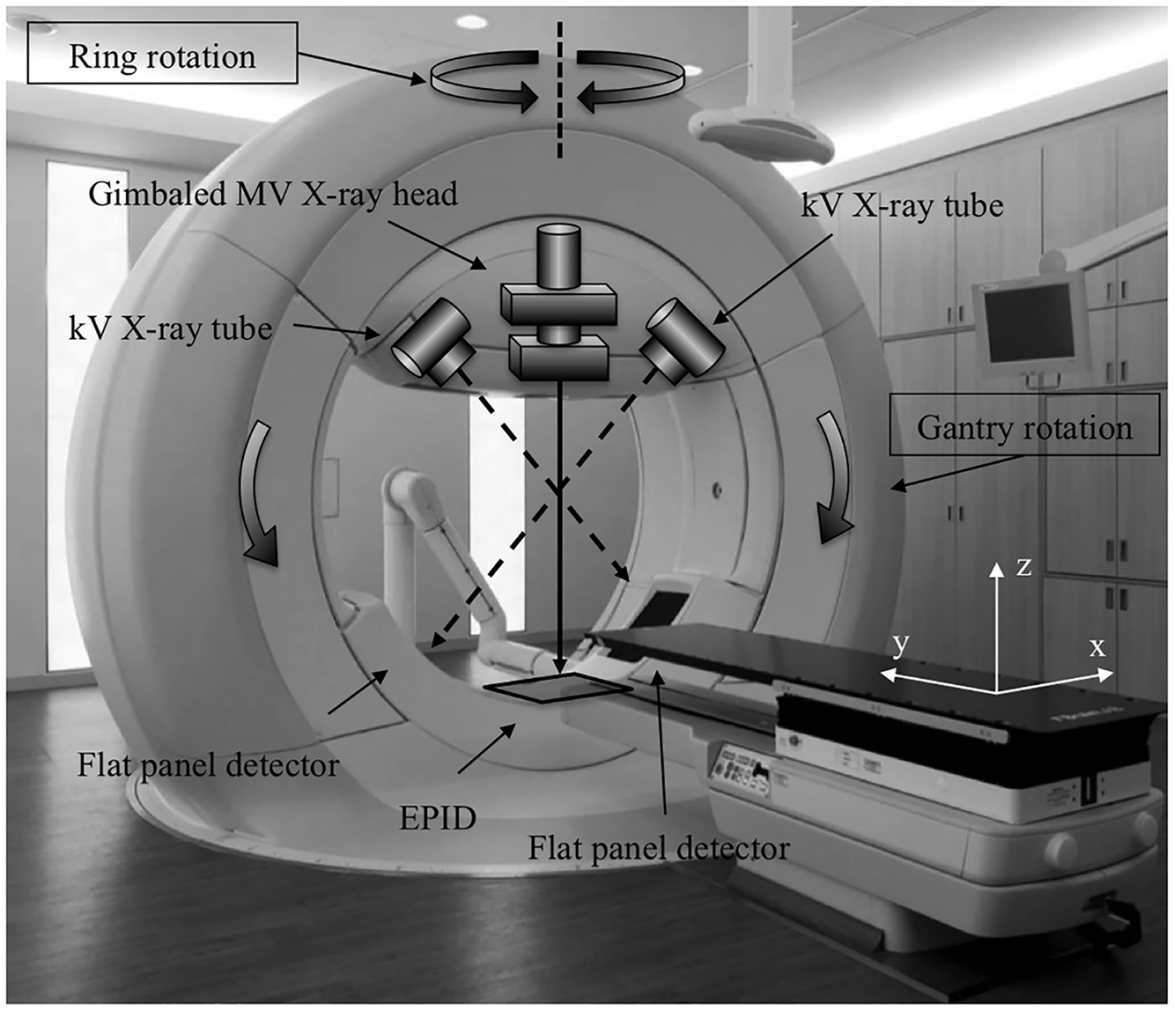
The Vero4DRT system with dual implementation of the kV imaging system and electronic portal imaging device (EPID). Exposure by non-coplanar MV beams was enabled by gantry and ring rotation. Dynamic tumor motion tracking was conducted through exposure to the gimbaled MV X-ray head. The lock-on system was used to correct sagging by the X-ray beam axis.
